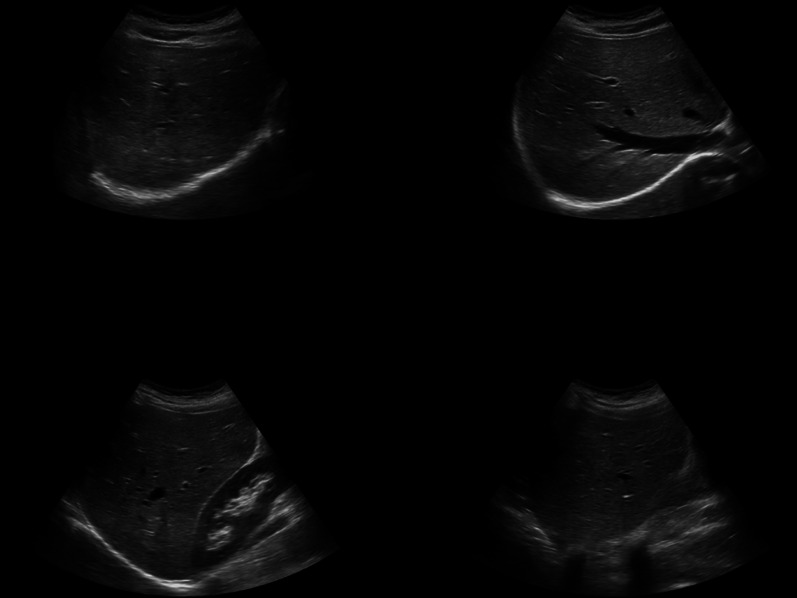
Example stimulus in Experiment 1a: collage of a liver. *Note* The collage contains two transverse and two longitudinal ultrasound views of the liver. In this example, the degree of hepatic steatosis is 2 (*Normal-mild*)
